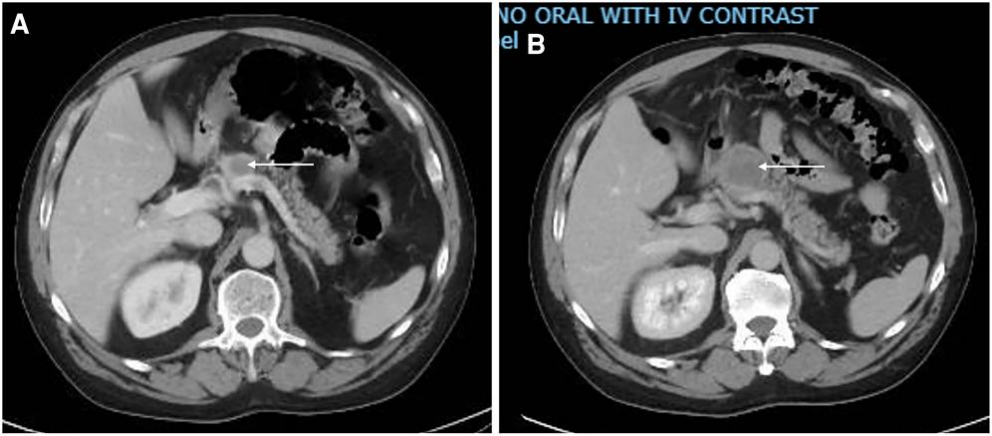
CT scan of the abdomen showing the 1.7 cm pancreatic mass (white arrow, panel A) decreased from 3.3 cm (white arrow, panel B).
